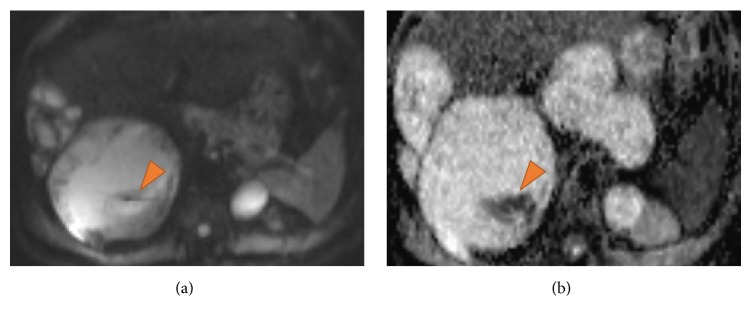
(a) DWI *b* = 50 and (b) ADC mapping at the levels of the liver hydatid cysts and renal cyst show higher signal intensity of the renal cyst at DWI, suggesting a simple cyst. There is also an area of water motion restriction identified as a focus of high signal intensity at DWI and low signal on the ADC map (arrows).
